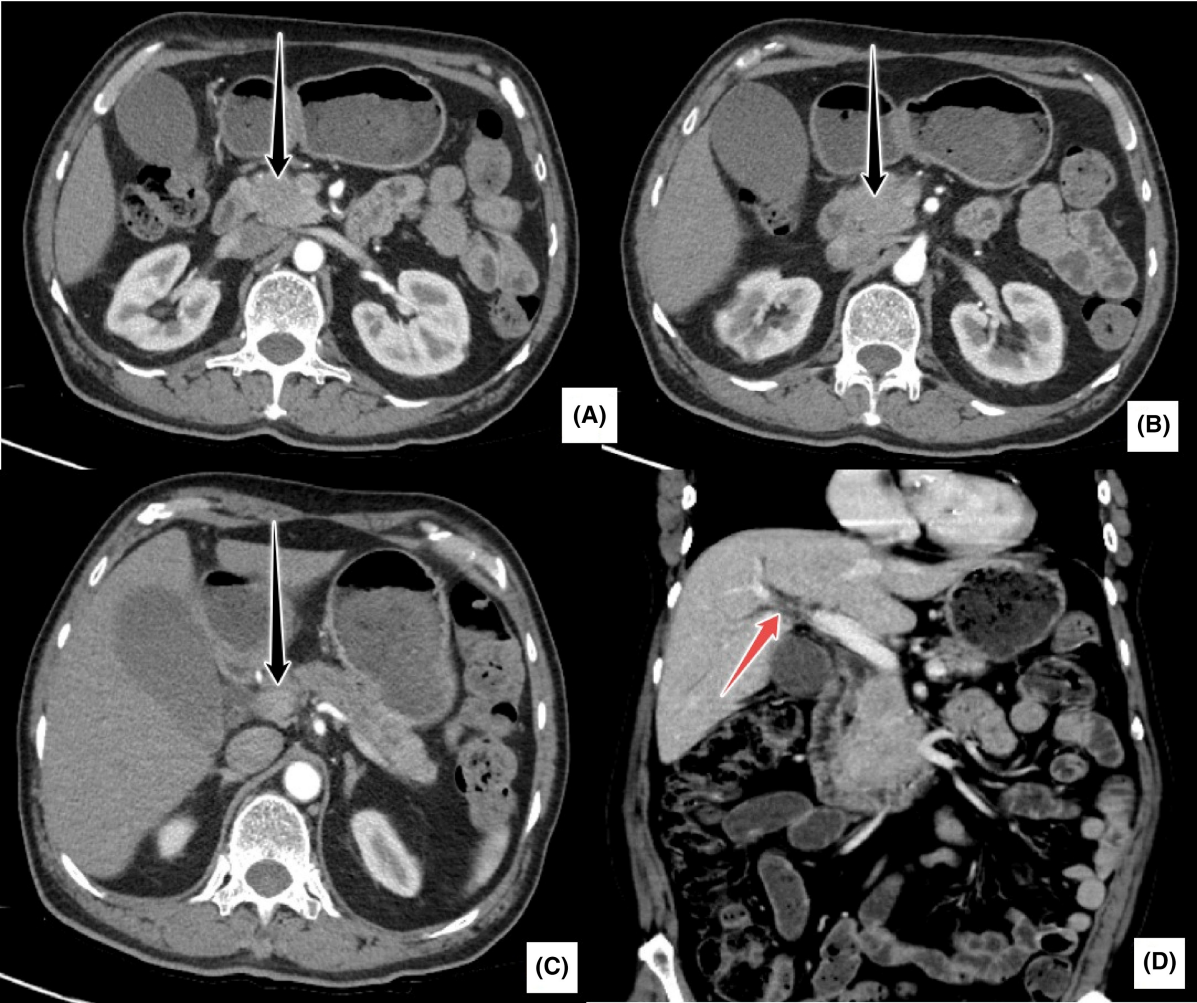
72‐year‐old male with the indication of pancreatic mass. Serum IgG4 levels were elevated at 2220 mg/L. (A–C) Images from abdominal contrast‐enhanced CT (CECT) showing a large mildly enhancing, lobulated, soft tissue mass involving the head and uncinate process of the pancreas (black arrows). (D) Note that despite a large pancreatic head mass, there is only minimal intrahepatic biliary dilatation (red arrow).
